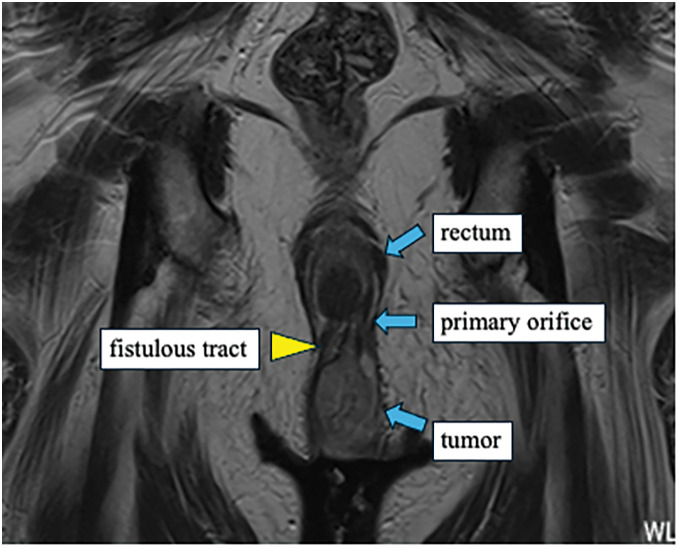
Pelvic MRI demonstrating a 56 × 21-mm tumor connected to the rectum via a fistulous tract (arrowhead).
